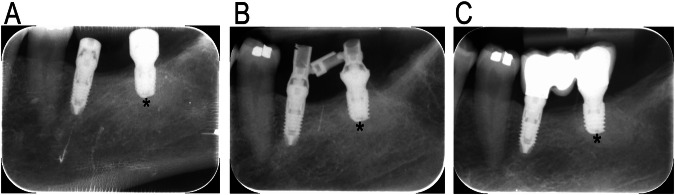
Clinical case. Periapical radiographs showing the marginal bone level at insertion (A), loading (B) and 12-month (C). (*) denotes the test implant.
